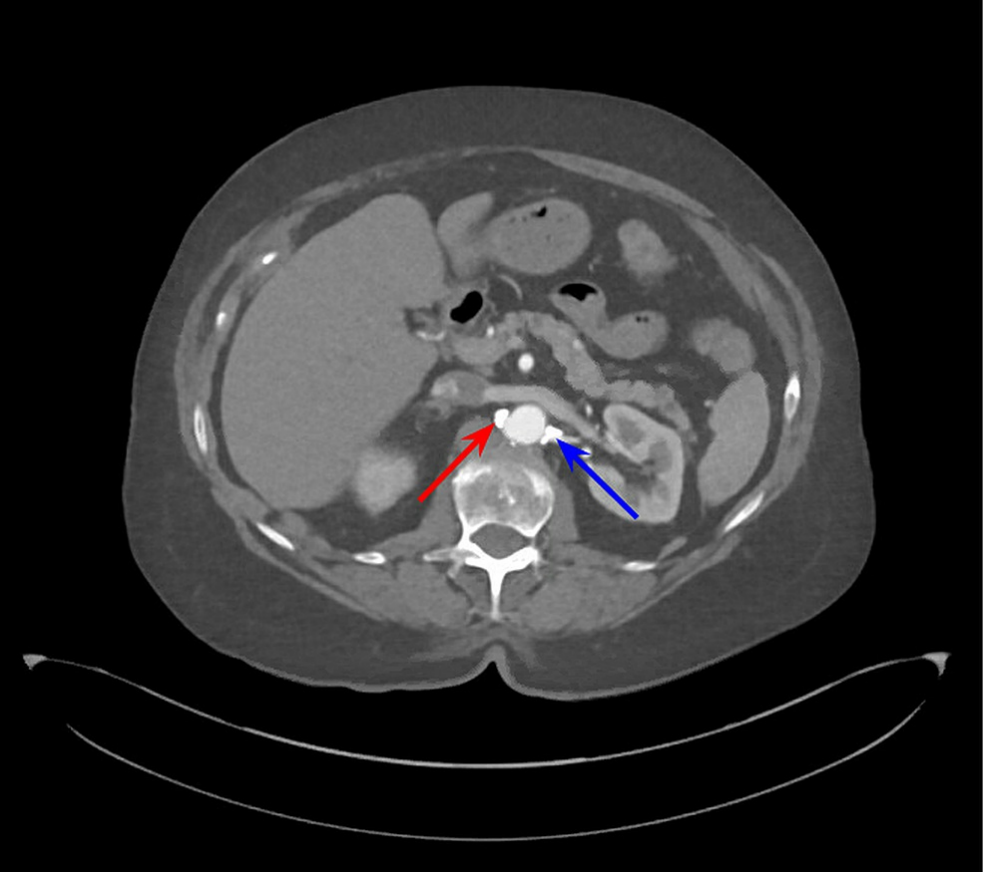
CTA abdomen demonstrating a high-grade stenosis of the right renal artery origin (red arrow) from aorta and >50% stenosis of the left renal artery (blue arrow) CTA - Computed tomographic angiography.
